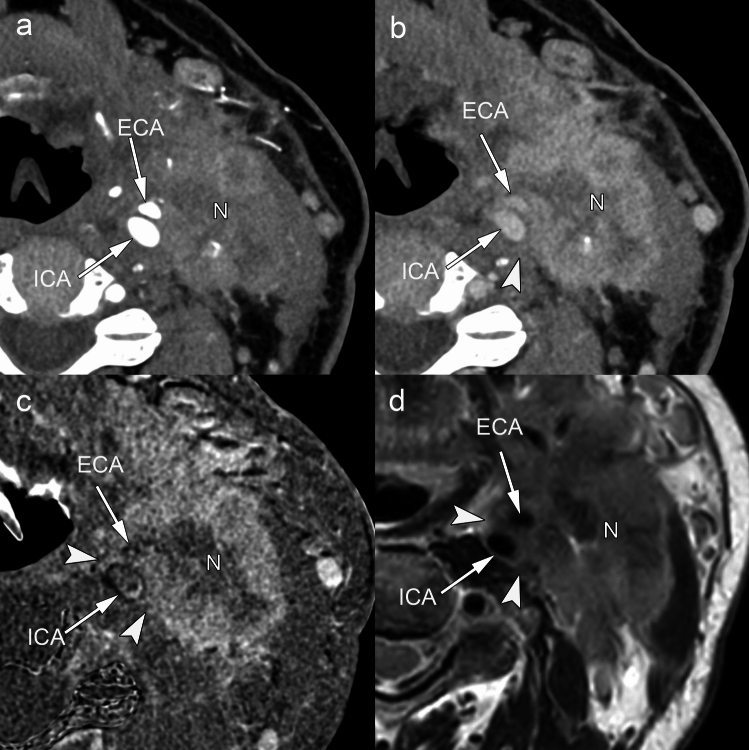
iENE Grade 3 with carotid artery invasion in a 74 year-old man with hypopharyngeal carcinoma. a Ultra-high-resolution early phase and b delayed phase show tumor extension to the left carotid bifurcation with near-complete encirclement of the external carotid artery (ECA). c Ultra-high-resolution subtraction iodine image clearly delineates the extent of tumor invasion. d T2-weighted MRI (3 mm slice thickness) confirms ECA encirclement by tumor more than 270°, meeting the criteria for probable carotid invasion and resulting in treatment modification with induction chemotherapy. ICA: internal carotid artery. Imaging parameters: a-c UHR-CT; SHR mode, 1024 × 1024 matrix, 1 mm slice thickness, DLR. d 3 T MRI; axial T2-weighted sequence, 3 mm slice thickness
